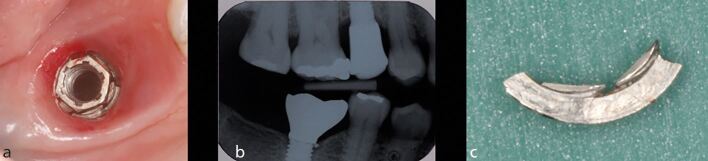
a) This patient presented with a loose implant crown in the right mandibular first molar site. The implant was placed 15 years previously, while the second molar tooth had been extracted two years ago. The patient had signs of attrition associated with parafunction. b) The associated radiograph showed a small radio-opaque fragment mesial to the implant head. Upon removal of the crown, multiple fractures of the implant shoulder were identified. A number of factors may have contributed to the fracture: 1) loss of the second molar increasing loading on the implant; 2) the slightly distal implant placement leading to non-axial loading of the implant; and 3) parafunction. c) The loose fragment that was removed from the implant
